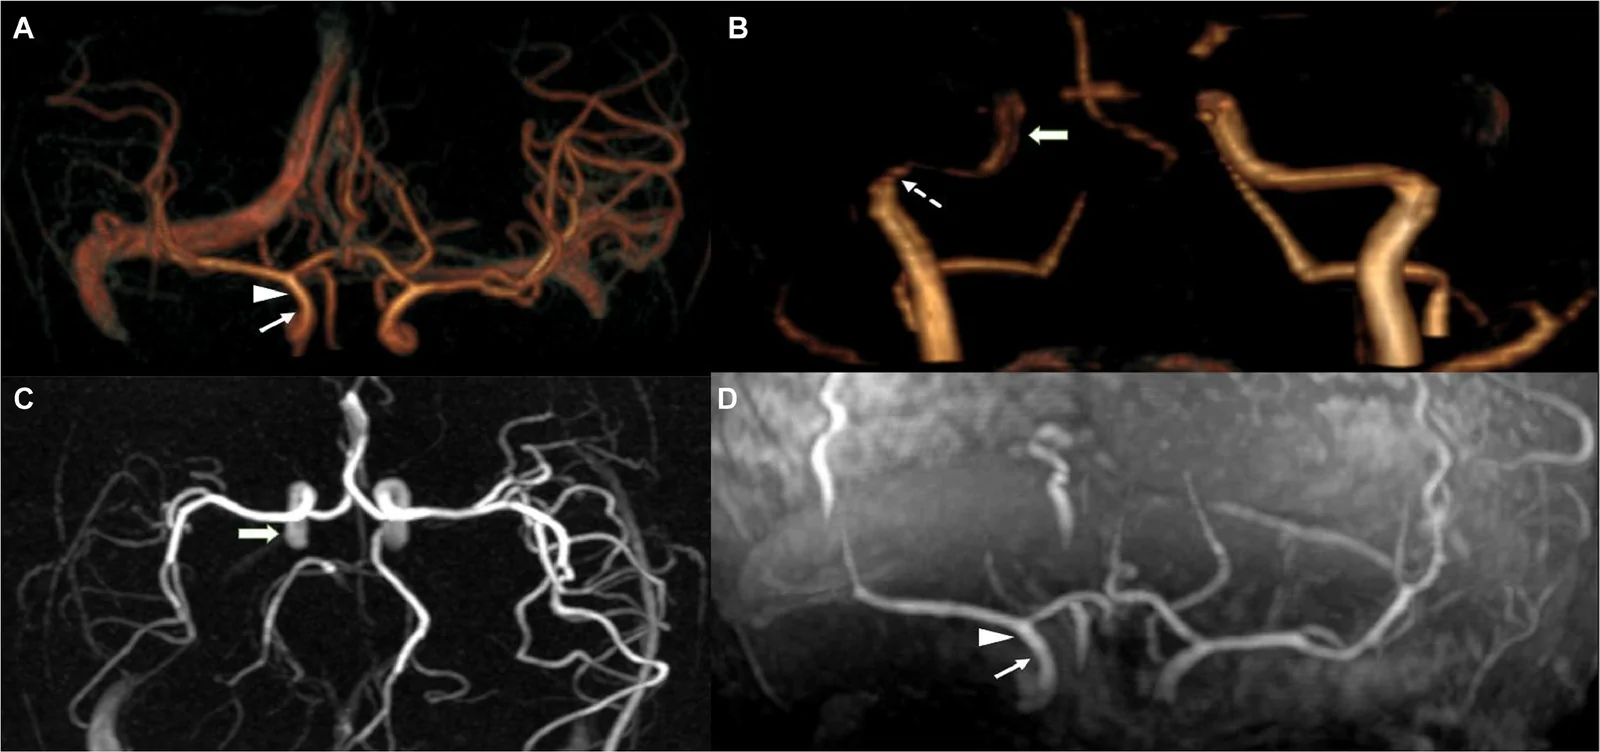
Three-dimensional (3D)-rendered MRI and TOF MRA (A-D) in a 6-year-old boy with NF1 show asymmetric long-segment narrowing of the right intracranial ICA, involving the petrous (*dashed arrows*), cavernous (*open arrows*), ophthalmic (*white arrows*), and communicating segments (*white arrowheads*). *ICA,* internal carotid artery; *MRI,* magnetic resonance imaging; *NF1,* neurofibromatosis type 1; *TOF MRA*, time-of-flight magnetic resonance angiography.
